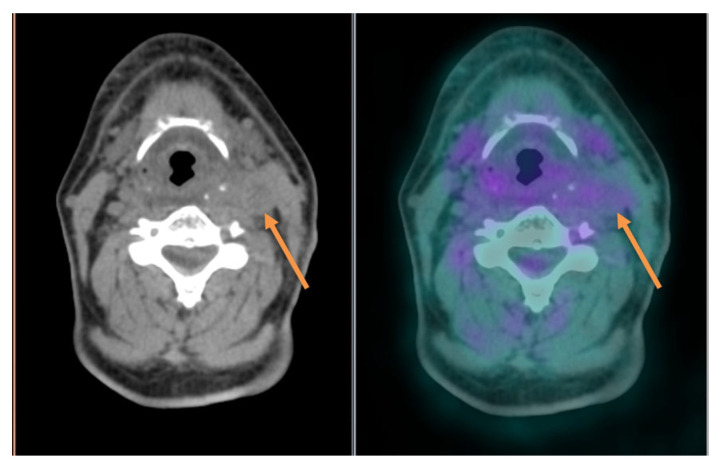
PET/CT images showing an enlarged and hypodense left cervical lymph node metastasis with no significant increase in ^18^F-FDG uptake due to necrotic changes (arrow).
